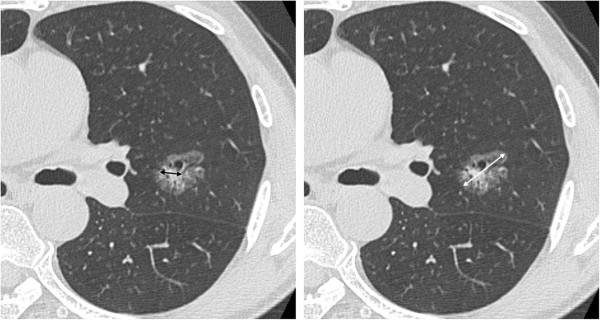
Example of the consolidation/tumor (C/T) ratio measurement on thin-section computed tomography. C/T ratio (%) = maximum diameter of consolidation (black arrow)/maximum diameter of tumor (white arrow) × 100.
